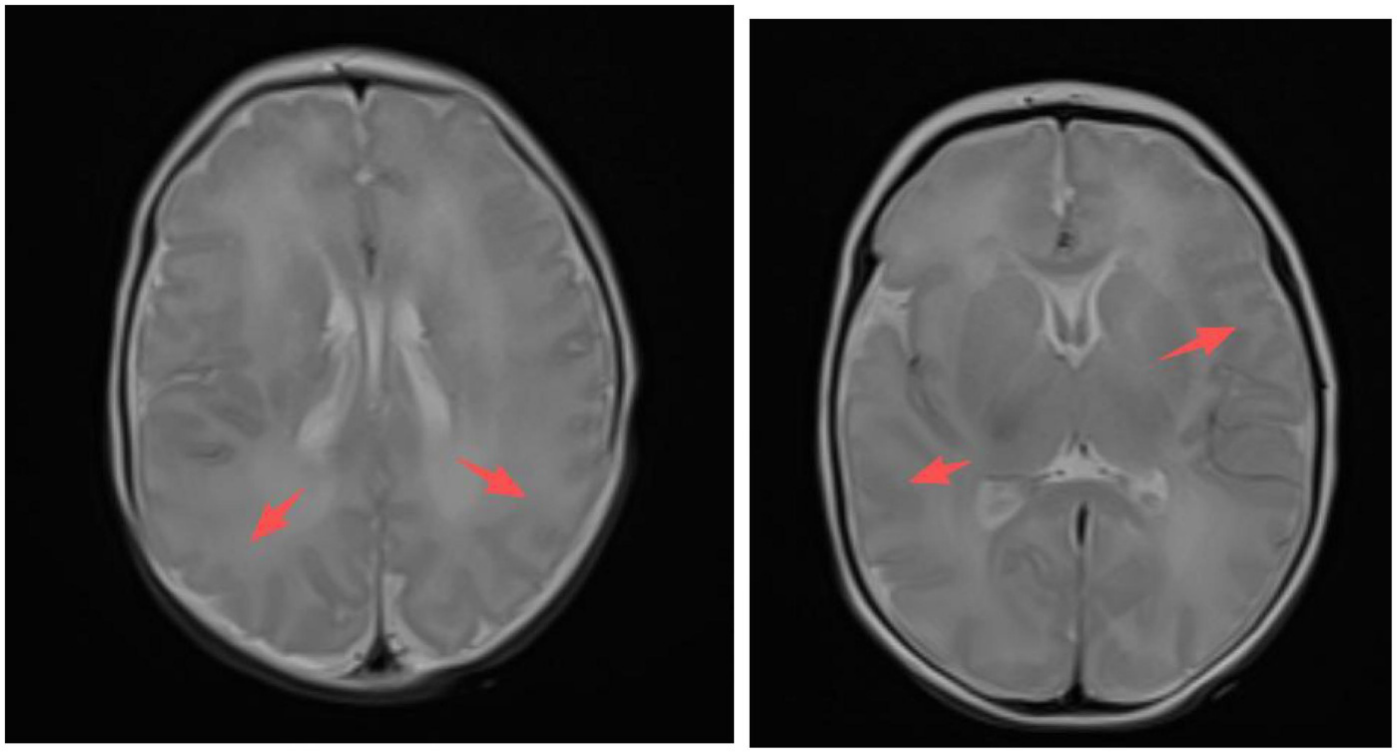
Abnormal brain MRI. Axial T2-weighted image demonstrates slightly swollen gyri with shallow sulci. Poor demarcation between gray and white matter. The normal distinct interface between cortex and white matter is blurred.
